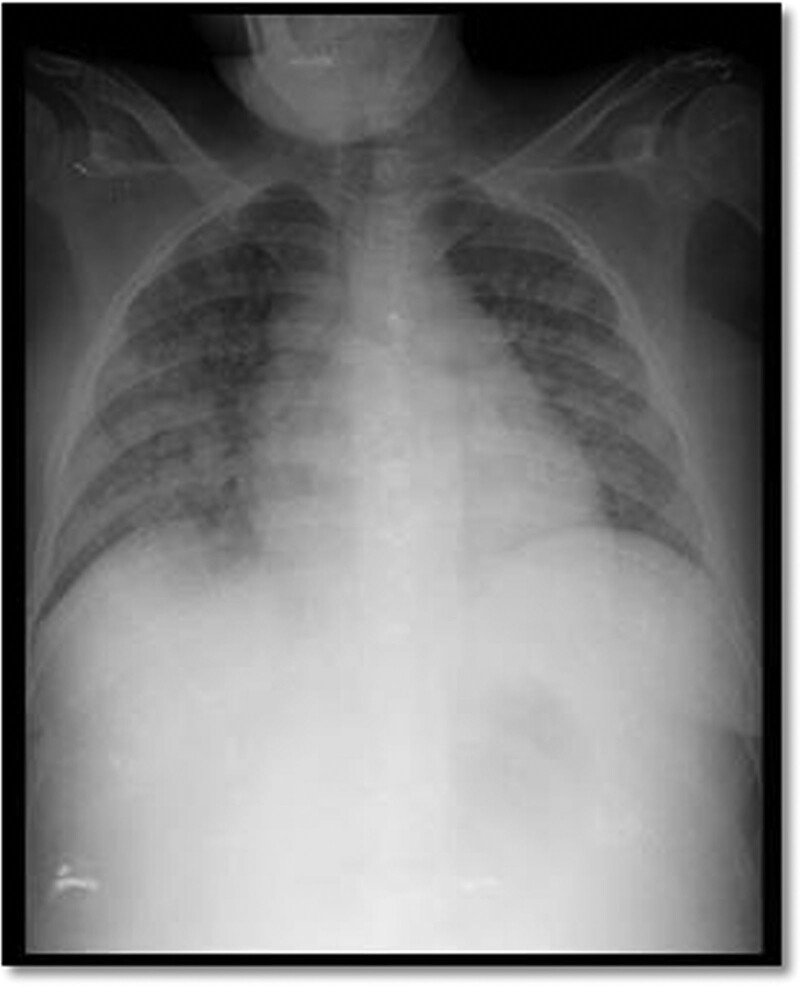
Chest radiograph showing pulmonary bilateral diffuse infiltrates.
